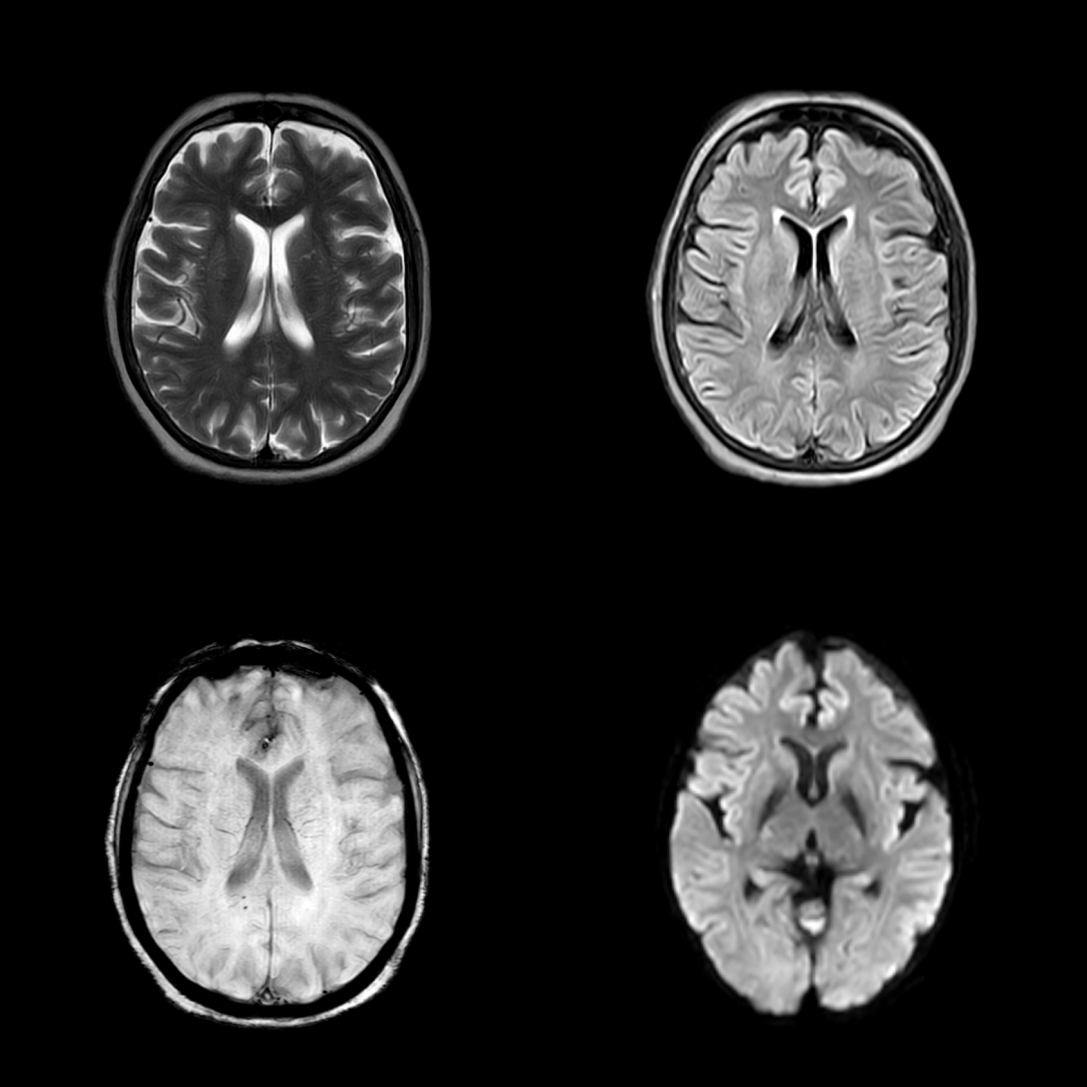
Magnetic resonance imaging (MRI) sequences, including T2-weighted imaging, fluid-attenuated inversion recovery (FLAIR), susceptibility-weighted imaging (SWI), and diffusion-weighted imaging (DWI), arranged in a clockwise orientation. The FLAIR sequence demonstrates features consistent with chronic small vessel ischemia; however, the overall MRI findings are inconclusive in the context of the clinical presentation.
